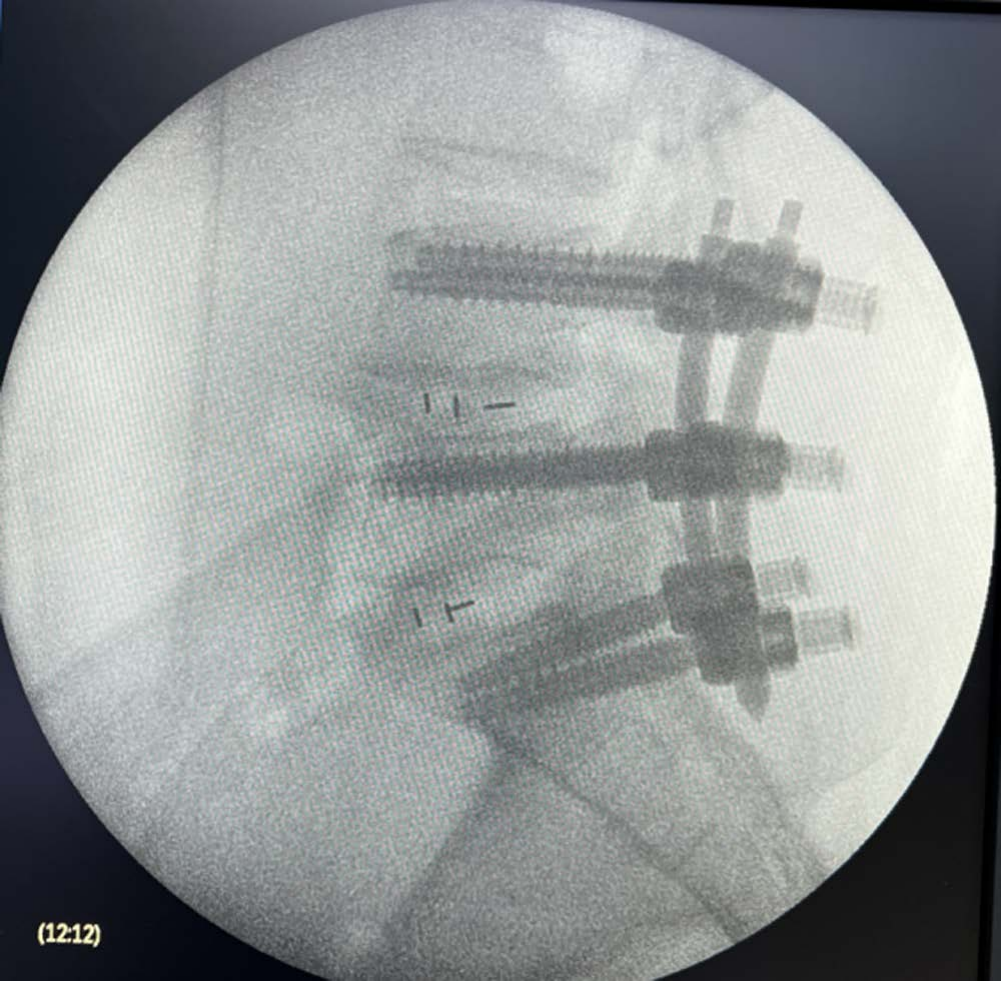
Spine radiograph with lateral view during the operation.
